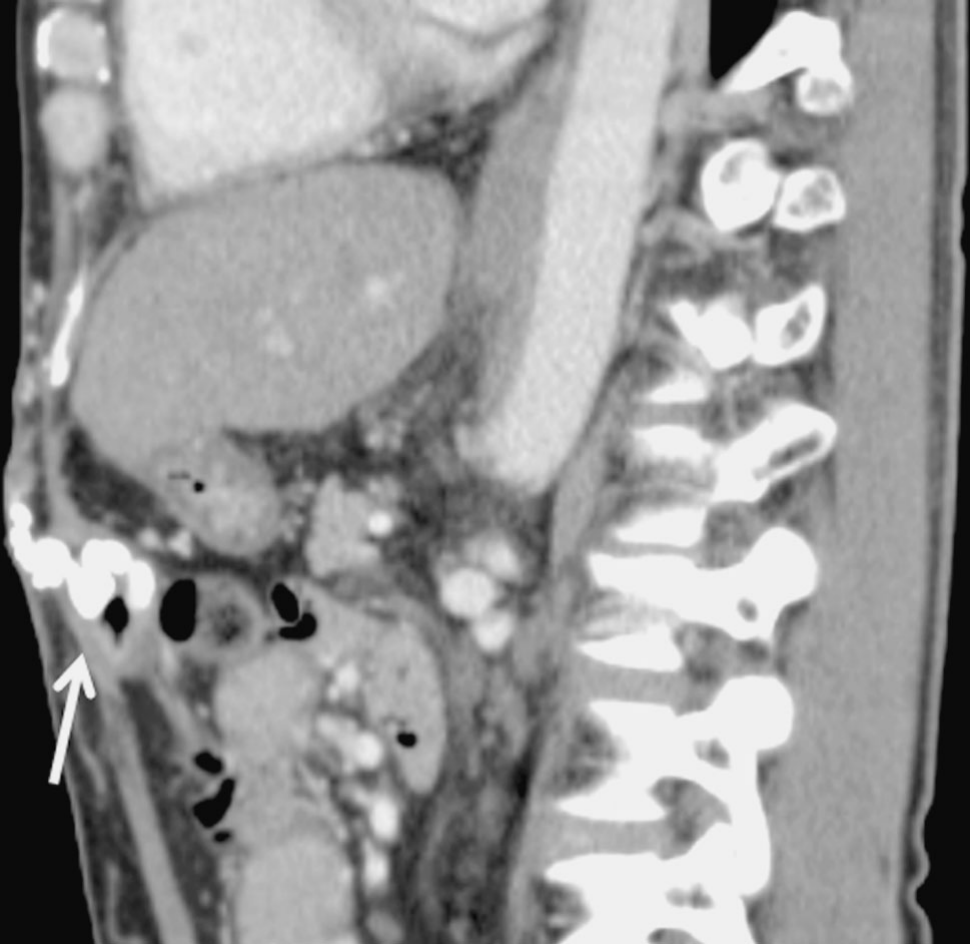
CT scan reveals the retention of the NBCA mixture in the mesenteric varices (*arrow*). Note the draining vein observed just after retrograde transvenous obliteration
